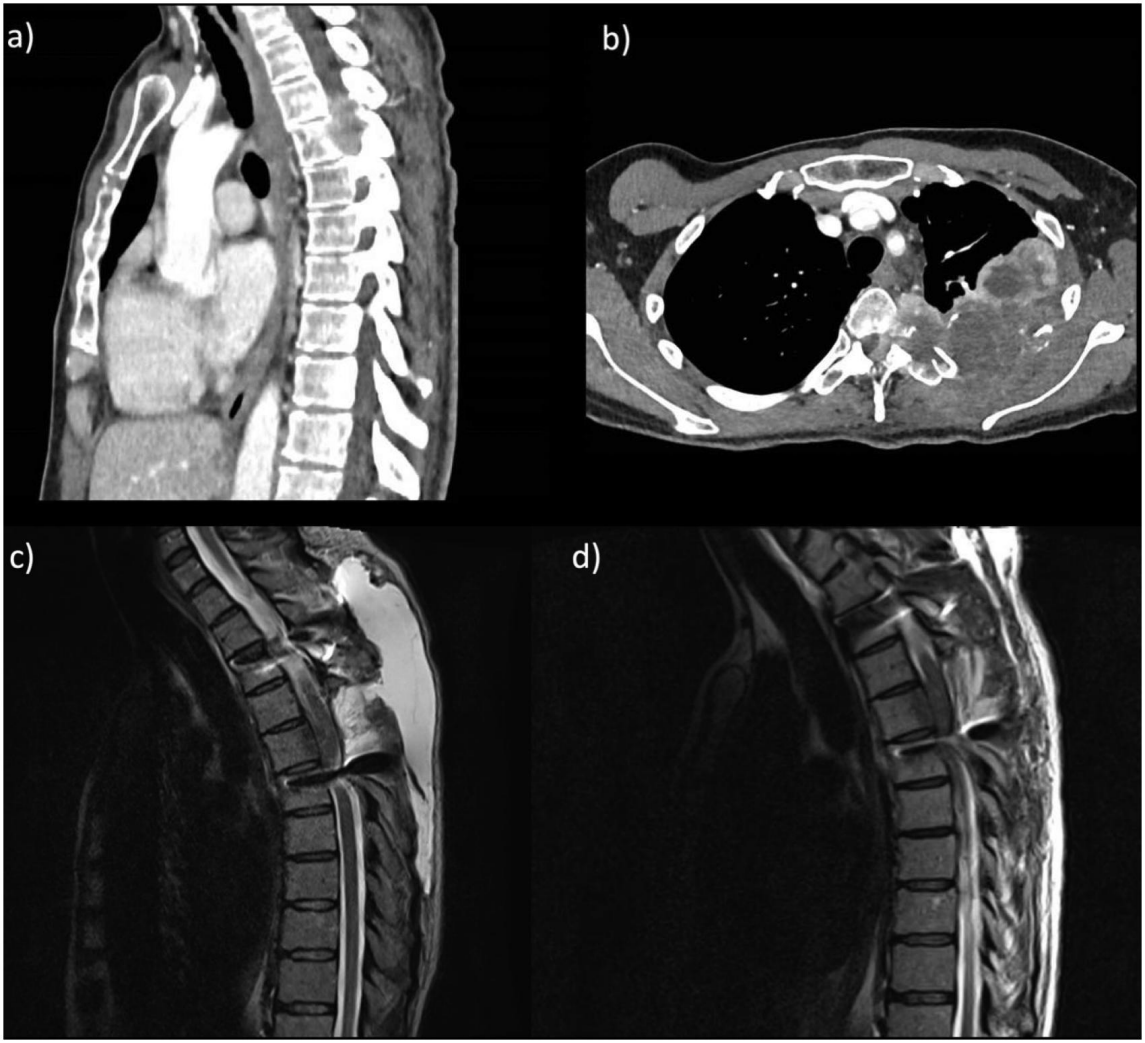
Case of malignant thoracic spine tumour with pre-operative CT images showing the paravertebral metastatic uterine leiomyosarcoma in sagittal (a) and axial (b) views. An MRI sagittal view of the CSF leak after the first operation is displayed in c), with a subsequent MRI after operative exploration, repair and CSF diversion shown in d).^28^^,^^29^
